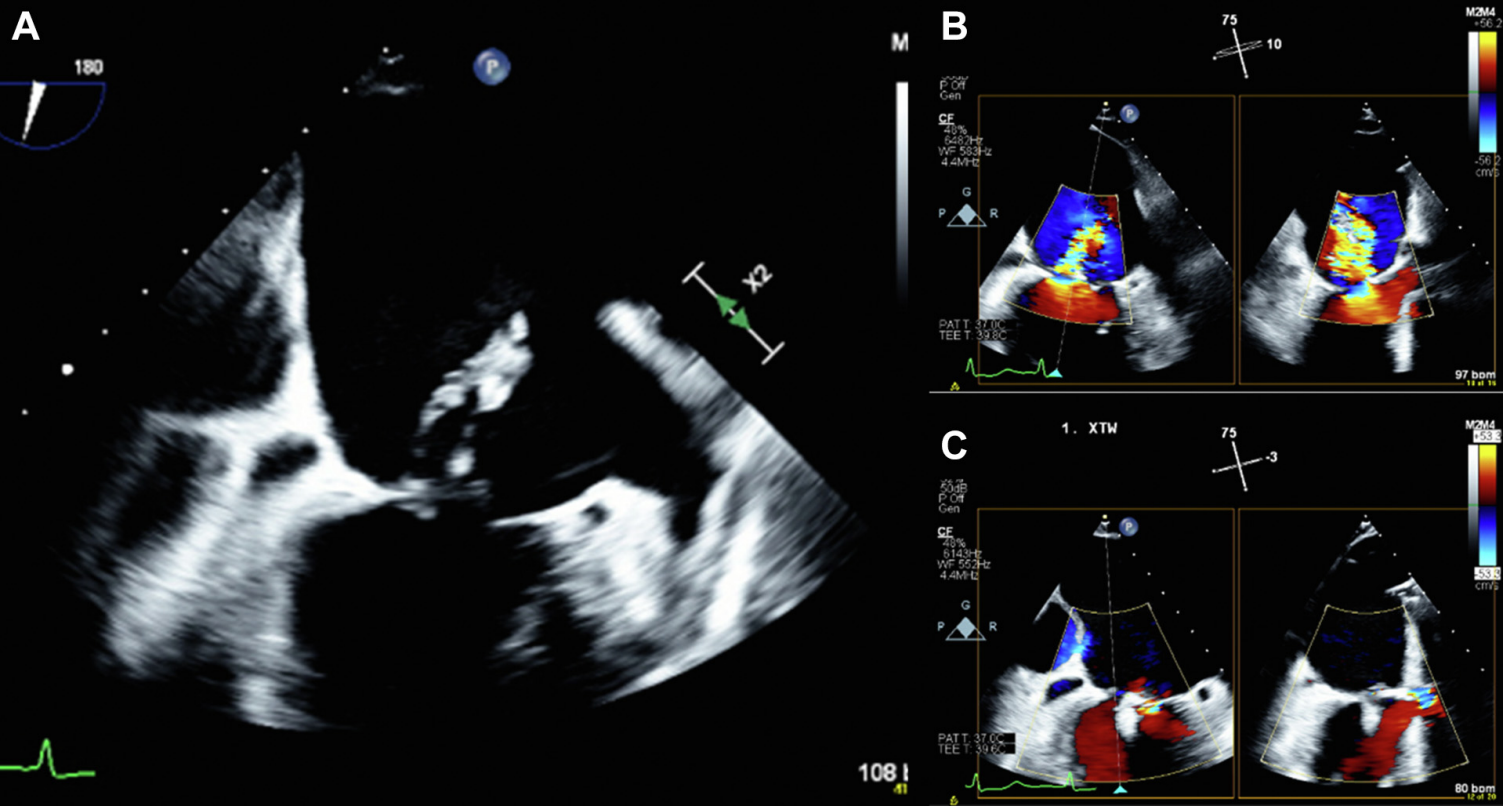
Postinfarction papillary muscle rupture with acute mitral regurgitation treated with transcatheteredge-to-edgerepair. (A) TEE: complete rupture of the posteromedial papillary muscle. (B) TEE: severe mitral regurgitation. (C) TEE: postprocedural trace mitral regurgitation. TEE, transesophageal echocardiogram.
